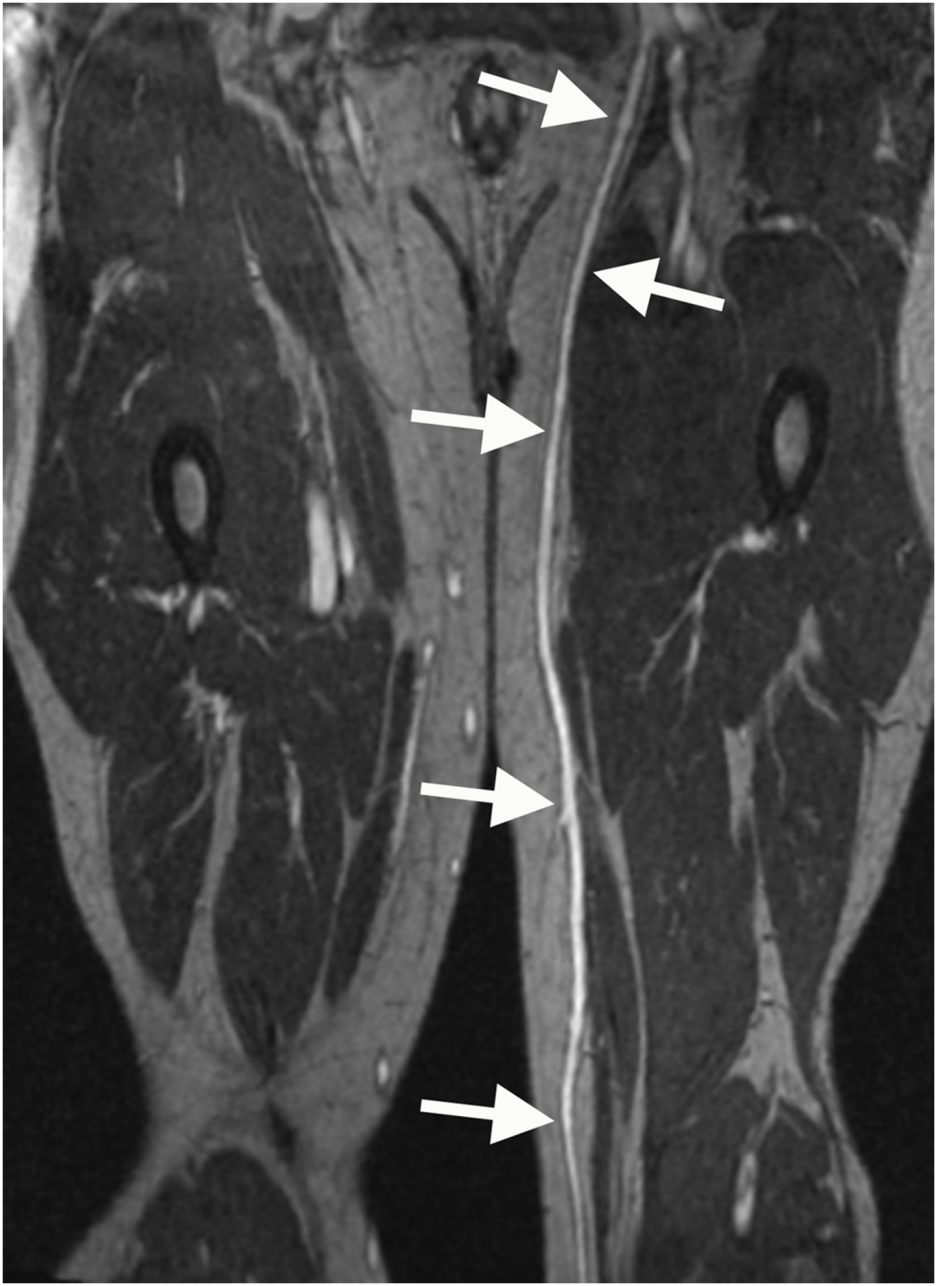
Multiplanar reformat of an MR-angiographic image of the great saphenous vein. The GSV displayed as a curved multiplanar reformat of high-spatial-resolution contrast-enhanced T1-weighted gradient-echo images during the steady-state of a 57 year old male patient suffering from PAOD stage III and thus being evaluated for bypass surgery.
